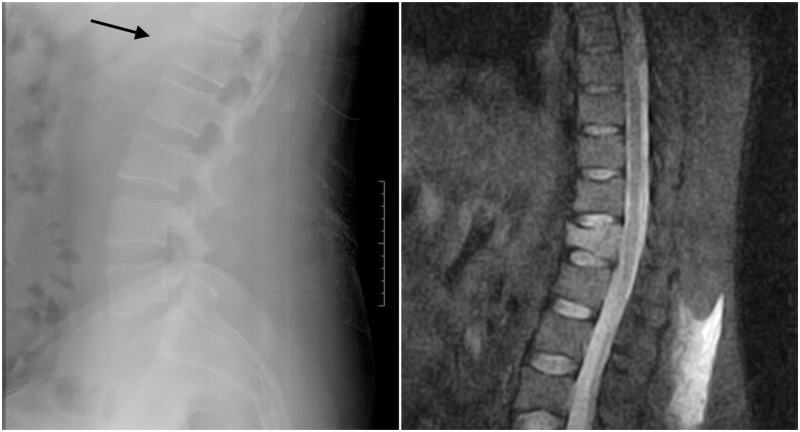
Lumbar compression fracture Left, lateral radiograph showing an L1 compression fracture (arrow). Right, magnetic resonance imaging (MRI) STIR displaying hyperintensity of the L1 vertebral body indicating an acute compression fracture. STIR - Short-TI Inversion Recovery.
